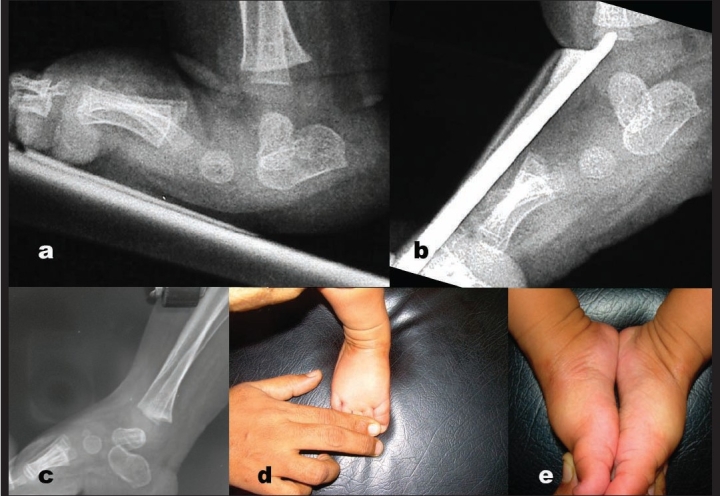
a) Lateral X-ray right foot in a child with congenital vertical talus shows irreducible talonavicular joint. b) Stress plantar flexion view of the same foot shows fixed plantar flexed talus. c) Follow up X-ray of the same foot shows reduction of talonavicular joint with the track of k-wire in talar body. d&e) Clinical photograph showing good correction of foot deformity and comparison with opposite foot.
